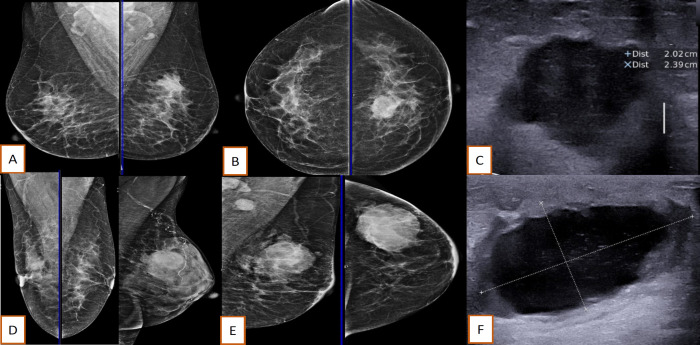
Mammo-sonographic images of Metaplastic Breast Carcinoma(MBC). A & B, Irregularly shaped high-density mass with indistinct margins (BIRADS 4c) in a mammogram of a 47 year old lady; C, Ultrasonography of the same lady depicting the mass to be hypoechoic with micro-lobated margins; D, Mammogram of two ladies showing iso-dense oval mass with pleomorphic calcification (BIRADS 4c); E, Mammogram of 41 year old lady with oval high-density circumscribed mass with partly indistinct margins with skin thickening and axillary nodes (BIRADS 4b); F, Ultrasonography of the same lady with complex cystic lesion
